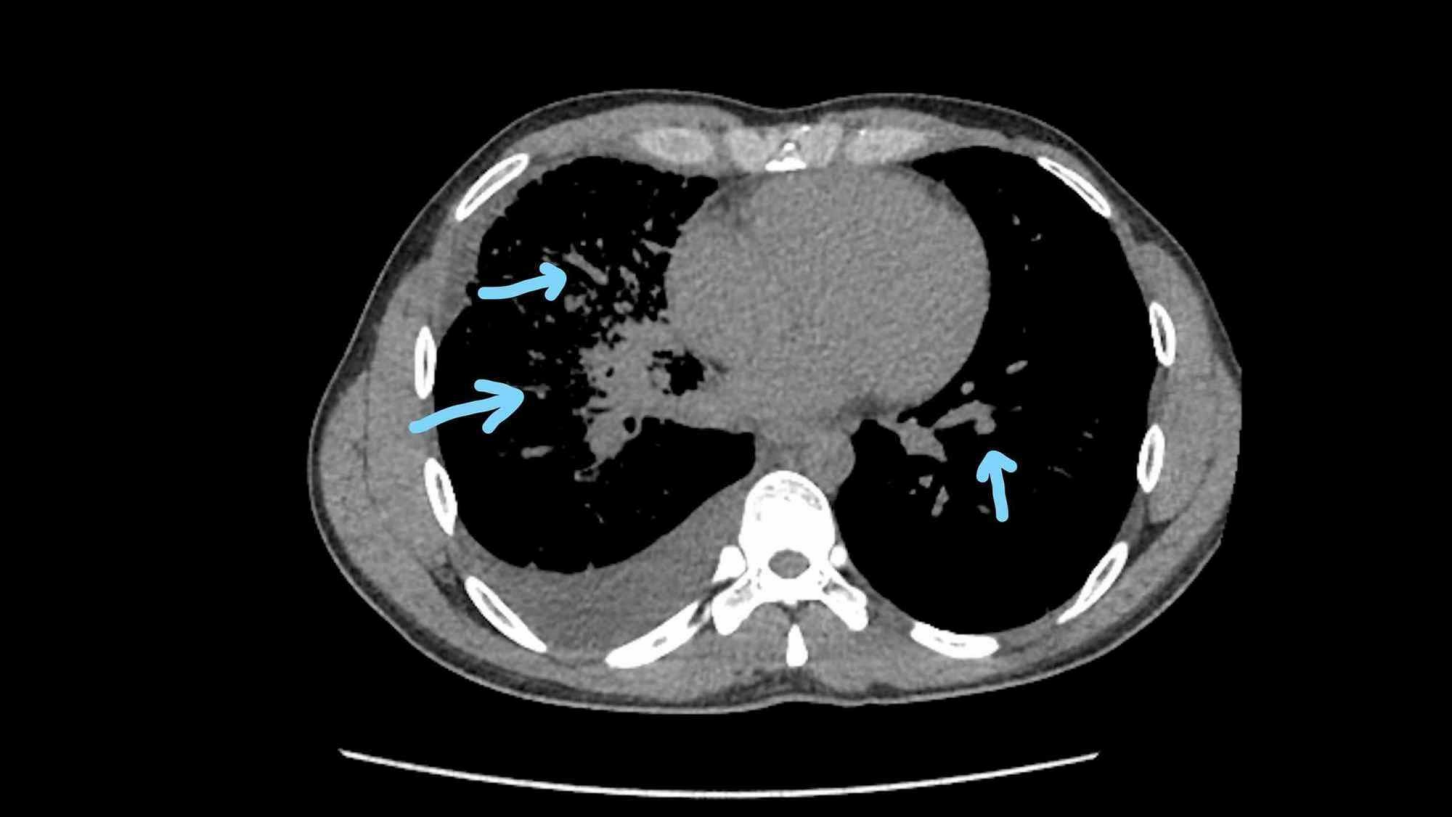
CT scan showing a diffuse bilateral miliary pattern Arrows pointing to bi-lateral multiple ground glass nodular opacities showing a miliary pattern
